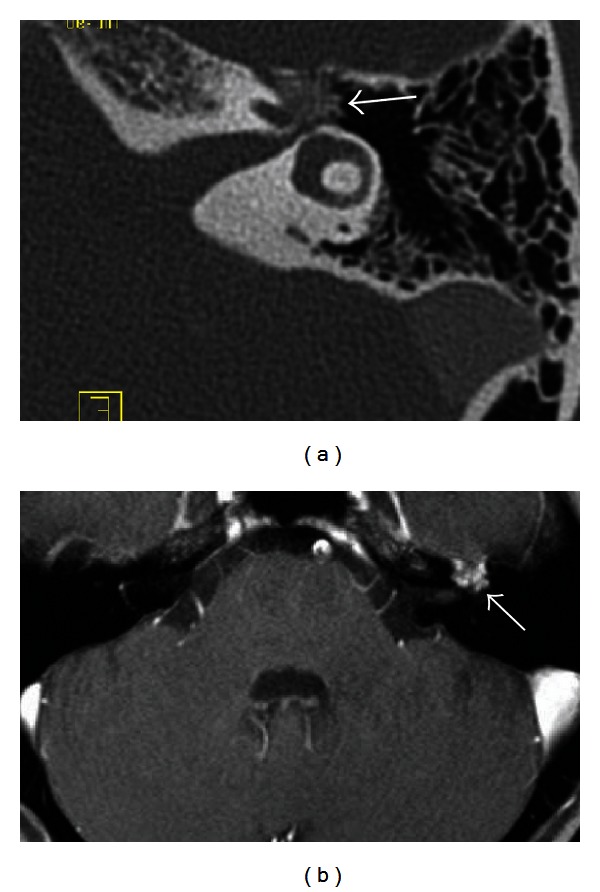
Facial nerve hemangioma. Axial CT (a) demonstrates a mass with trabecular bony matrix centered in the first genu of the facial nerve (arrow). Axial T1 postcontrast fat-saturated MRI (b) demonstrates the enhancing hemangioma (arrow).
